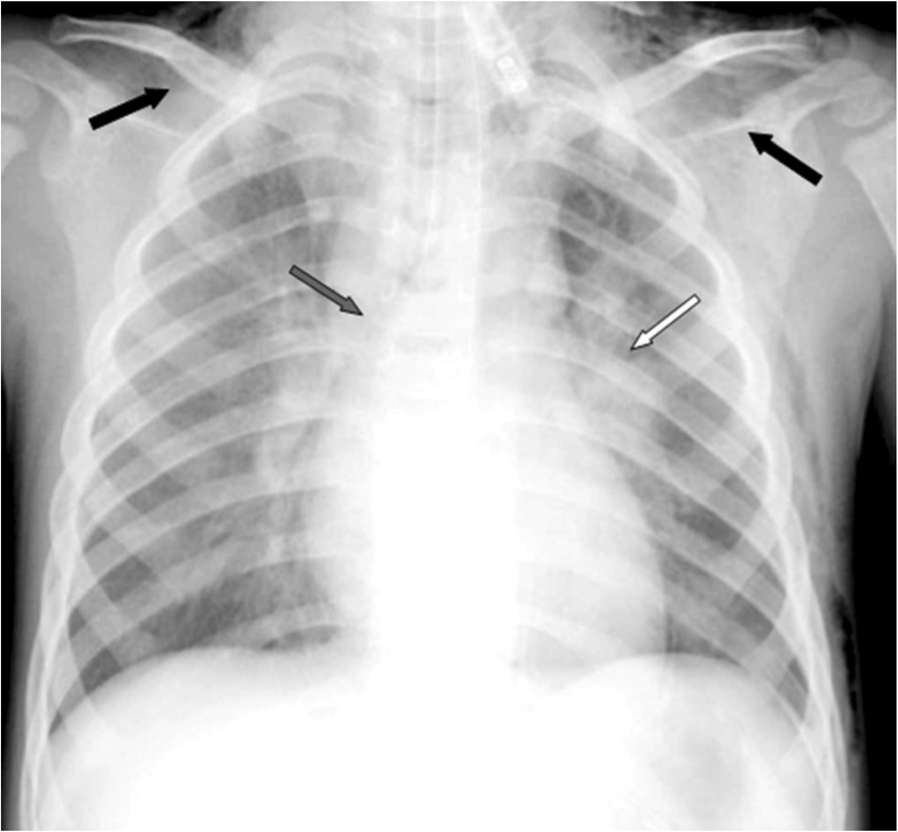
Chest X-ray showing a significant enlargement of the left lung hilum (white arrow) with pneumomediastinum (grey arrow) and subcutaneous emphysema (SE, black arrows).
